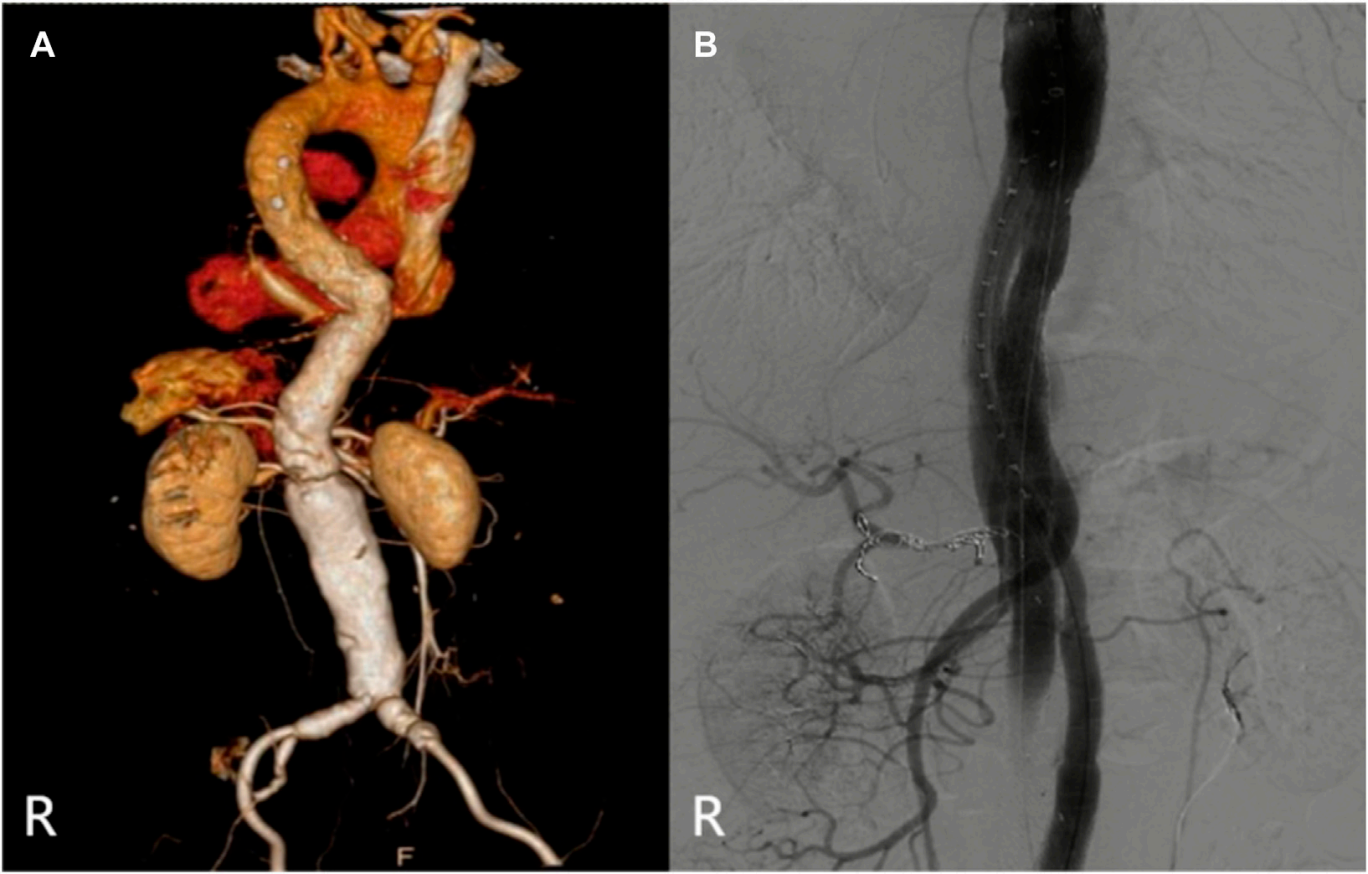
(Case 5) (A) Preoperative CTA. (B) Intraoperative imaging.
